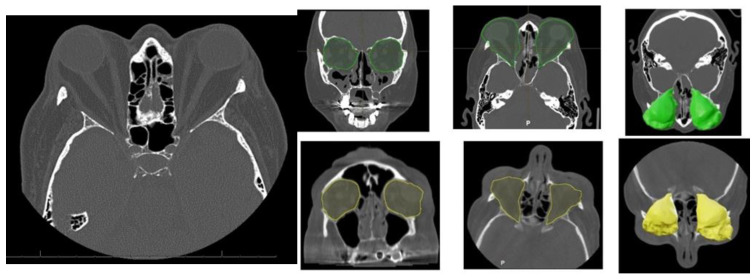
Characteristic orbital findings in Graves’ orbitopathy. Axial and coronal CT images showing hallmark features of Graves’ orbitopathy, including bilateral enlargement of extraocular muscles (notably the inferior and medial rectus), with sparing of the tendinous insertions. Increased orbital fat volume and anterior displacement of the globe (proptosis) are also visible. These changes contribute to compressive effects on the optic nerve and are commonly used for diagnostic and preoperative planning purposes. Preoperative intra-orbital volume shown in green; postoperative volume in yellow.
